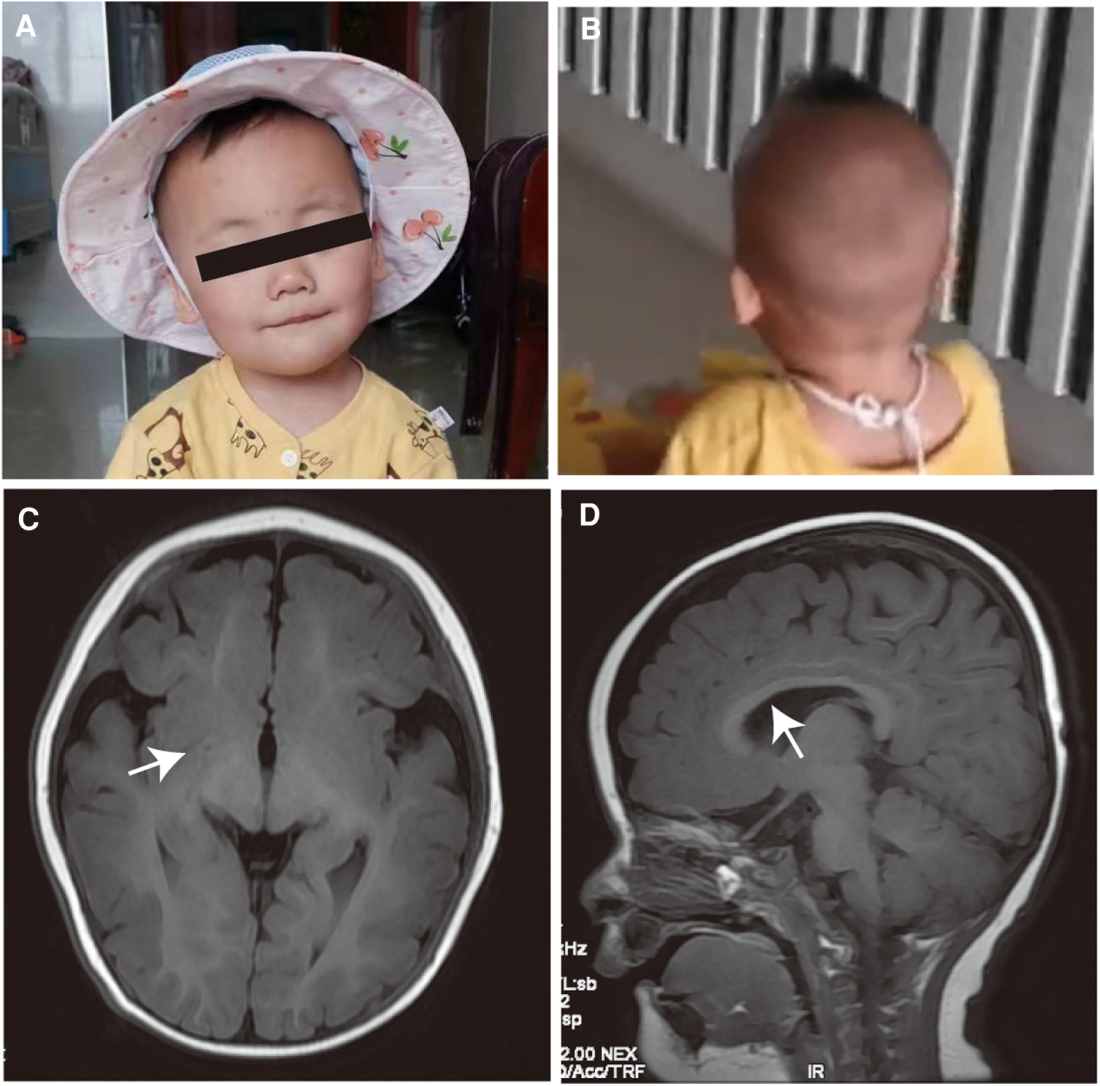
Clinical features. (A,B) Facial features of the affected individual. She has a short, upturned nose with a sunken bridge, thin lips, and flat occiput. (C,D) The brain MRI results in an infant aged 1 year 3 months. The white arrows show delayed white matter myelination and the thinly developed splenium of the corpus callosum.
